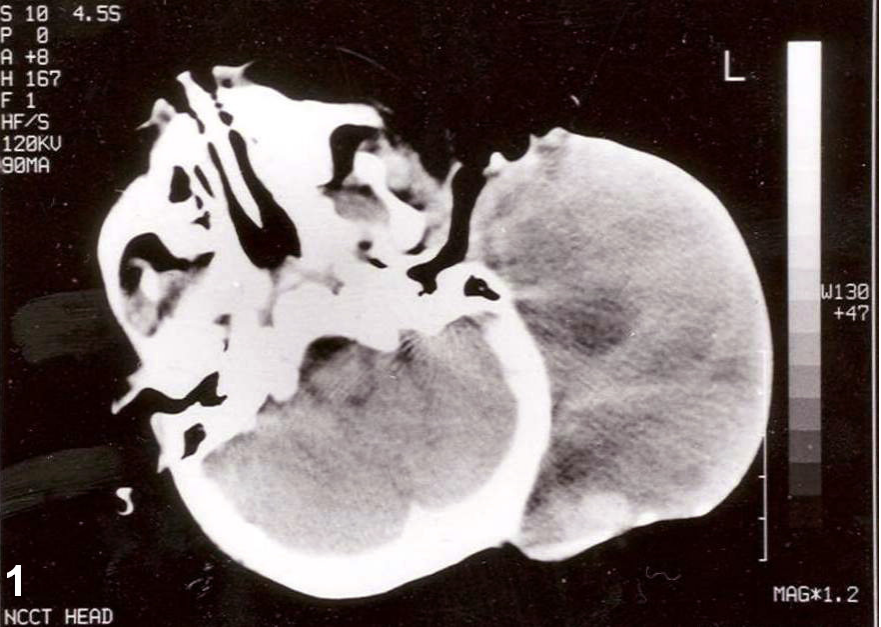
Non-contrast computed tomography of head showing the hypoechoeic mass without any intracranial extension.
